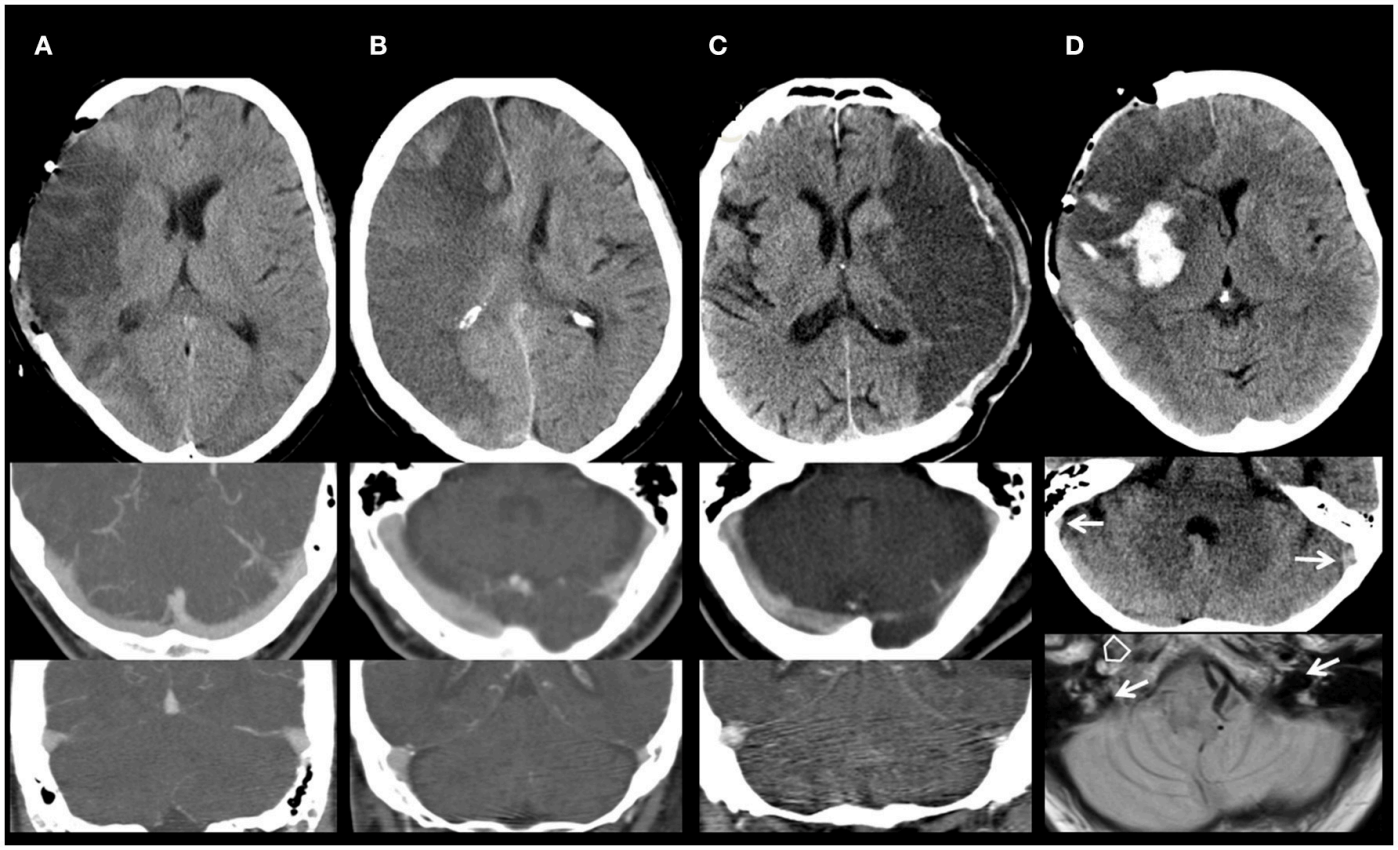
Imaging of cerebral venous drainage. (A) Normal appearing venous drainage. Non-contrast computed tomography (NCCT) of a 60-year-old man with a large right middle cerebral artery (MCA) infarction after decompressive surgery. Symmetric venous drainage by both ipsilateral and contralateral transverse sinus and internal jugular veins (CTA). (B) Abnormal contralateral venous drainage. NCCT of a 66-year-old woman with a space-occupying right MCA and anterior cerebral artery infarction. Hypoplasia of the contralateral transverse sinus and internal jugular vein (CTA). (C) Abnormal ipsilateral venous drainage. NCCT of a 60-year-old man with a large left MCA infarction who underwent decompressive surgery. Hypoplasia of the ipsilateral transverse sinus and internal jugular vein (CTA). (D) Abnormal ipsilateral venous drainage. Imaging of a 62-year-old woman with a large right MCA infarction, after decompressive surgery (NCCT; Upper). Hypoplasia of the ipsilateral transverse sinus and internal jugular vein. NCCT (Middle): Asymmetry of the sigmoid sinus, hypoplastic right, dominant left (arrows). MRI (proton density; Lower): basal scan close to the foramen magnum. Open arrow: occluded right internal carotid artery (missing flow void). Filled arrows: dominant left internal jugular vein (IJV), hypoplastic right IJV.
